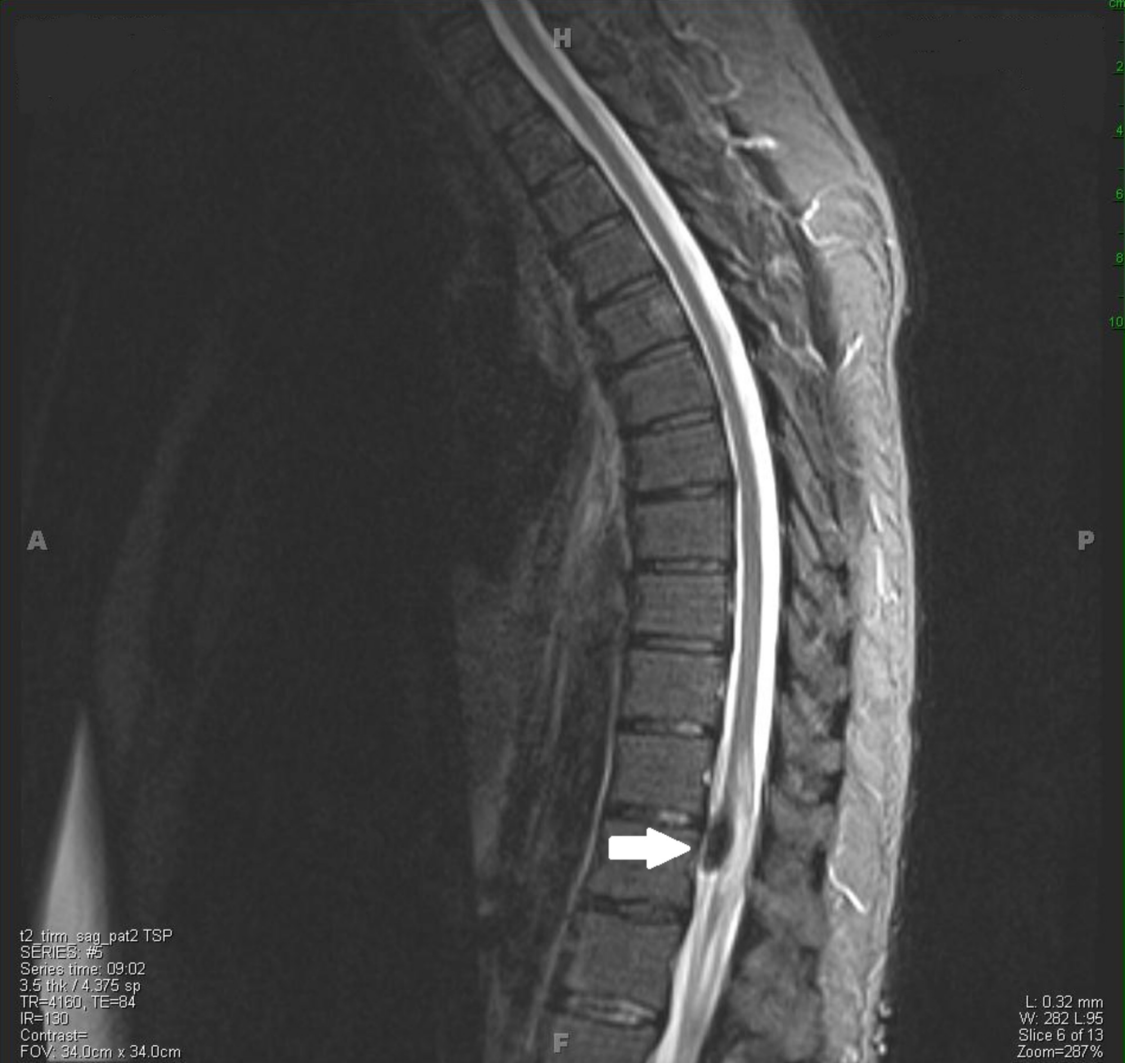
T2-weighted sagittal MRI displaying the intradural lesion.
